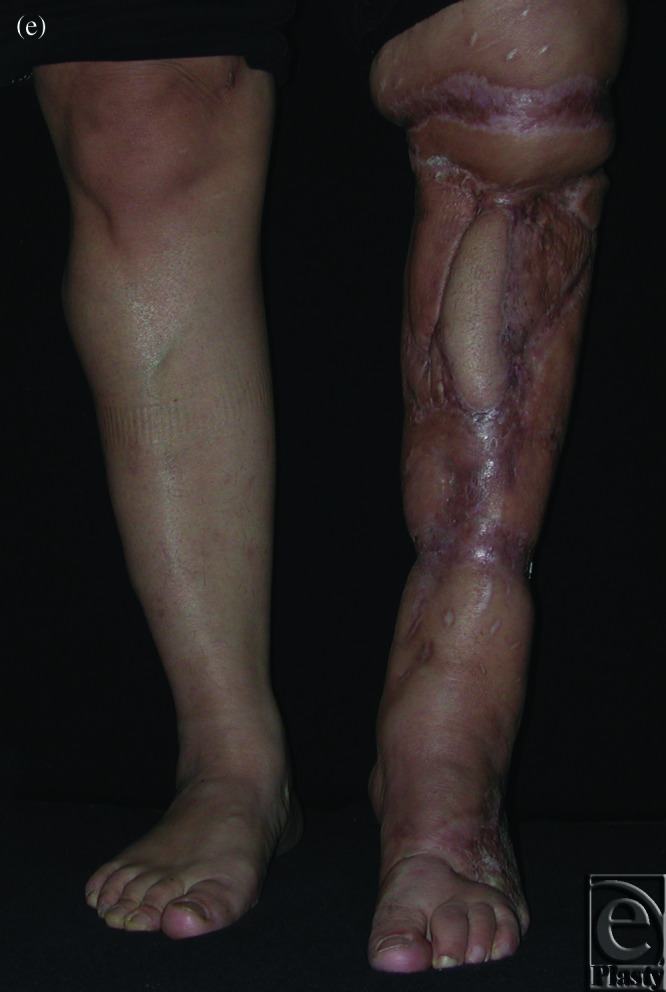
Case 2: Trauma of the lower leg. (*a*) Correction of the deformity by applying the Ilizarov device, (*b*) Preoperative x-ray, (*c*) Fibula osteocutaneous flap, (*d*) External fixation, (*e*) The 1-year postoperative view, and (*f*) A 1-year postoperative x-ray.
